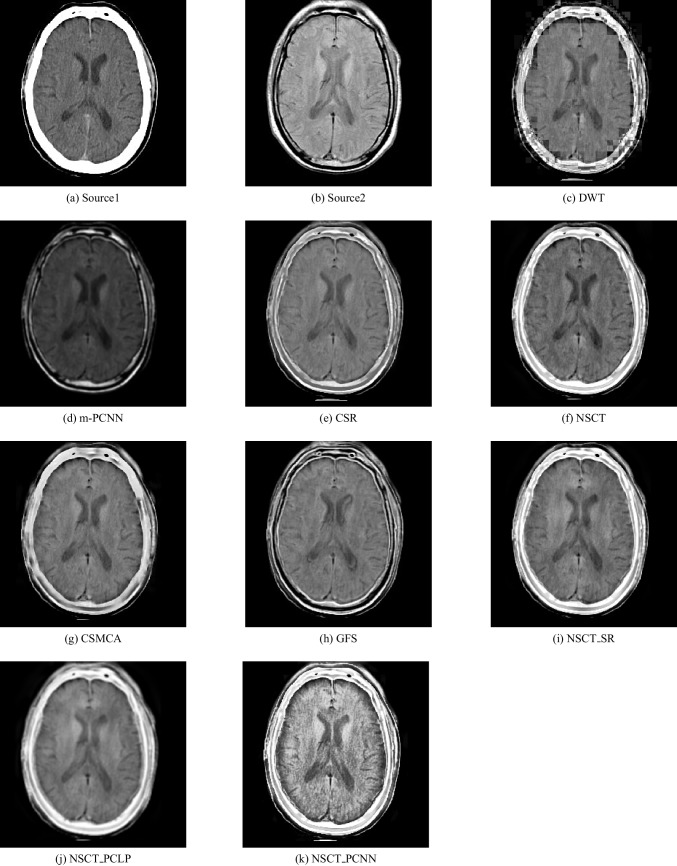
Fusion result of (a) CT and (b) MR-Gad using (c) DWT method, (d) m-PCNN method, (e) CSR method, (f) NSCT method, (g) CSMCA method, (h) GFS method, (i) NSCT-SR method, (j) NSCT-PCLP method, and the proposed method (k) NSCT-PCNN
